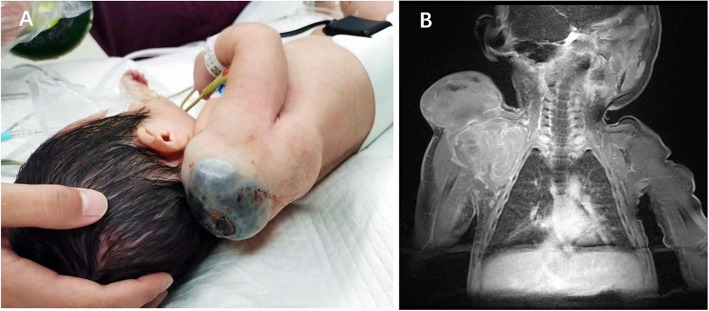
Giant nodular mass on the right shoulder with black skin surface and ulceration on the top of the skin, accompanied by a small amount of bright red blood exudation (a). MRI showing the localization of the tumor on the right shoulder and underarm with a lobulated appearance, surrounding the right scapula which was deformed (b)
